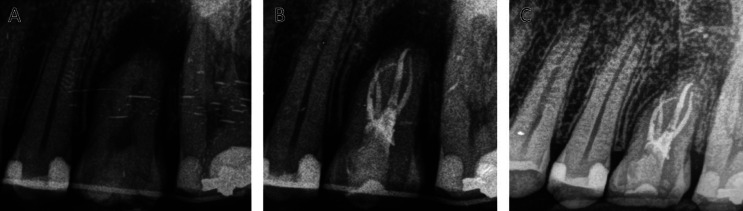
A. Periapical radiography after surgery. B. Periapical radiography and a root canal treatment after 7 days of the surgery. C. Radiographic control after 12 months of follow-up. PL conservation and bone formation around the root and interproximal bone maintenance can be observed.
